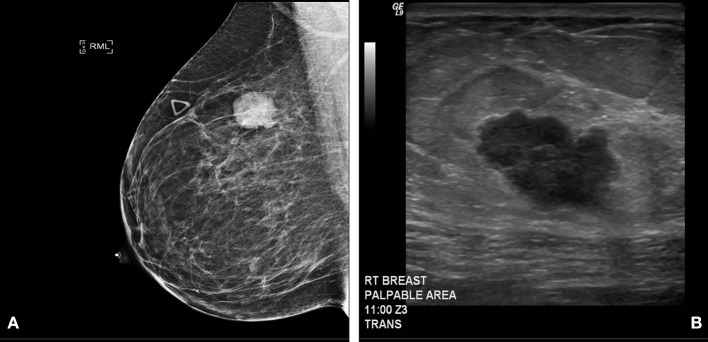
(A) Diagnostic Mammogram: Right medio-lateral oblique view showing an irregular high density mass in the superior central aspect of the right breast. (B) Ultrasonography of right breast and axilla: Irregular hypoechoic macro-lobulated mass in the superior central aspect of the right breast.
